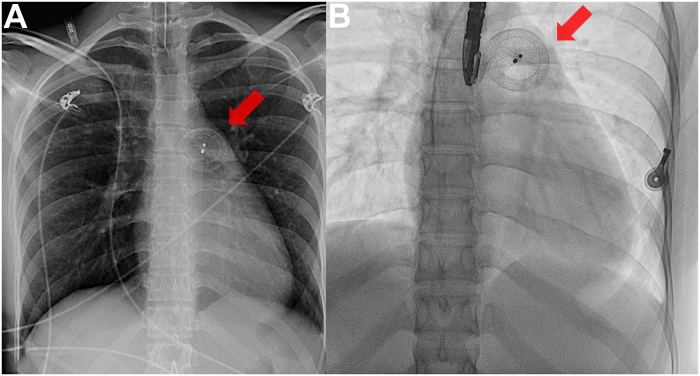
Chest Radiograph Prior Referral and Intraprocedural Baseline Fluoroscopy (A) Chest radiograph showing the 26-mm Amplatzer atrial septal occluder projecting over the bifurcation of the main pulmonary artery (red arrow). (B) Baseline intraprocedural fluoroscopy demonstrating the unchanged embolized position of the device (red arrow).
